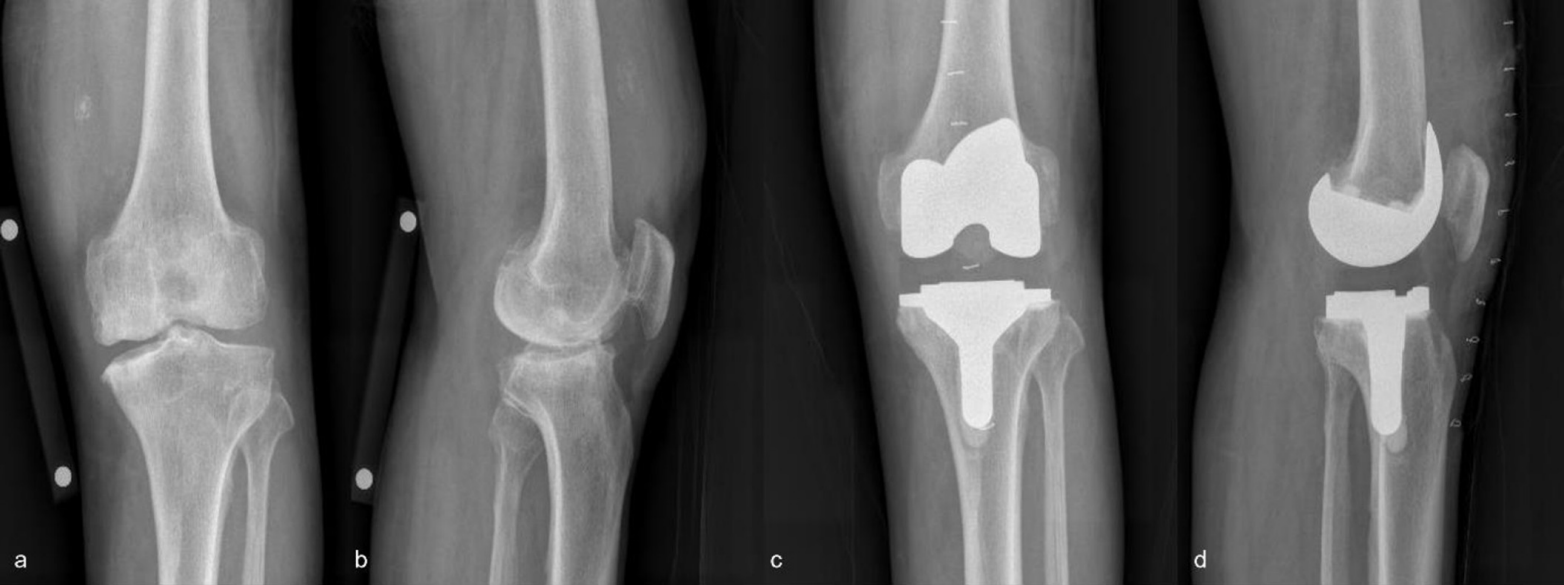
(a and b) Advanced osteoarthritis in a 67-year-old patient and (c and d) total knee replacement performed on the patient. A tibial posterior cortical bone breach is observed on postoperative radiography. No pain or signs of progression were observed on follow-up. The decision was made to not proceed with revision surgery.
